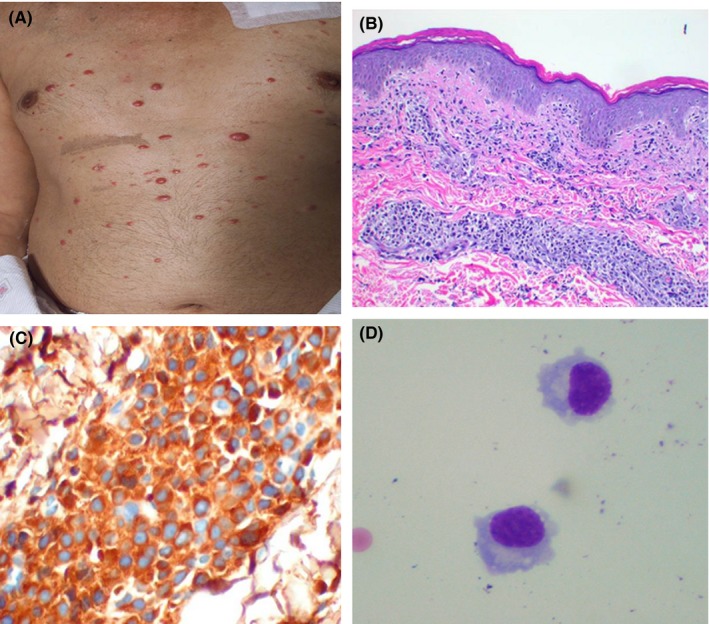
On the day of admission, the patient was found to have new right upper extremity weakness and a papular rash (A) on the chest. Biopsy of a chest papule showed infiltration of the skin by plasma cells (B) with kappa light chain restriction (C). Evaluation of the weakness including MRI and CT scan of the head showed no significant findings. Cerebrospinal fluid examination demonstrated the presence of multiple plasma cells with Kappa light chain restriction (D)
